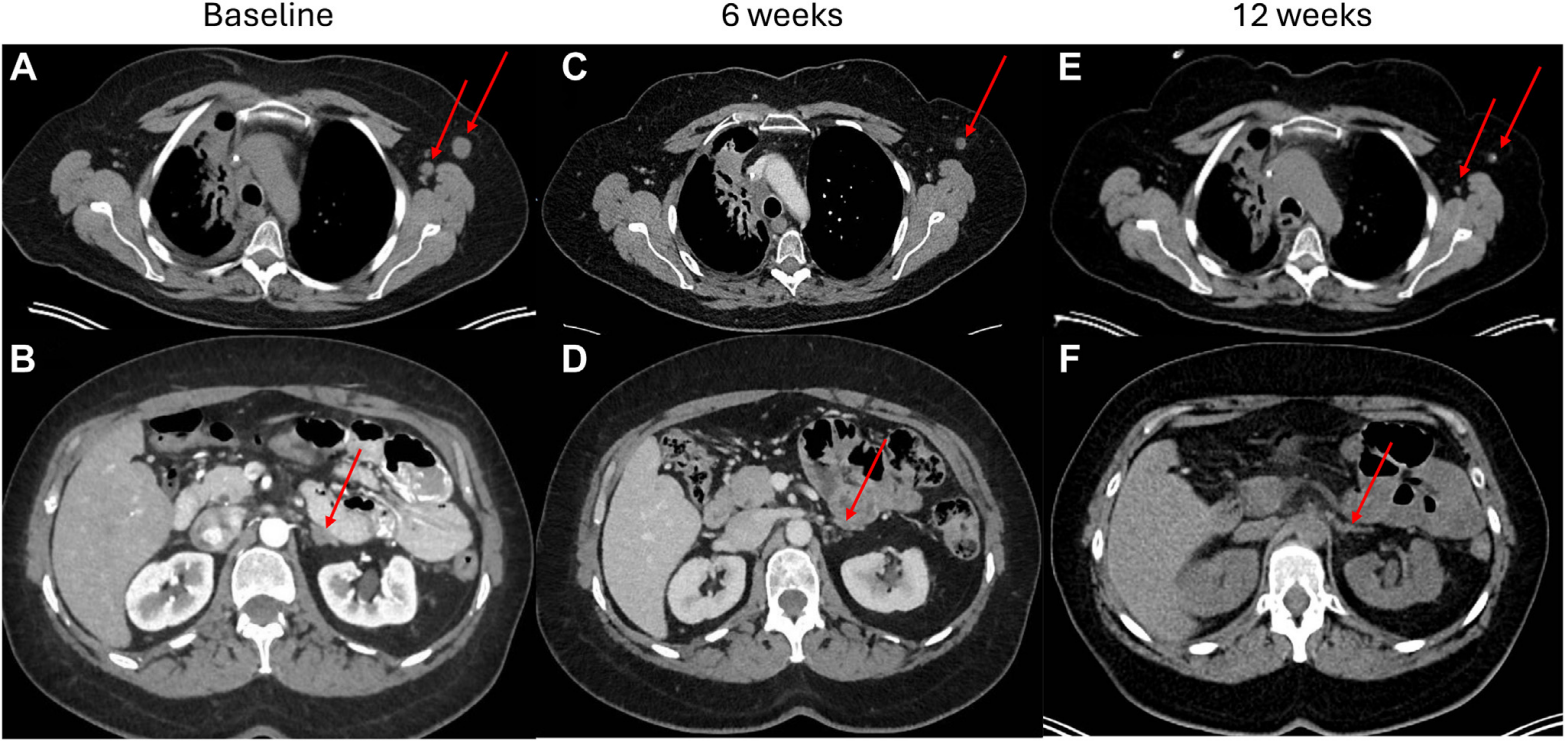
Baseline, 6-week, and 12-week imaging of key lesions revealing response. (*A*) Baseline, CT component of PET/Dotatate, enlarged left axillary lymph nodes; (*B*) baseline, CT abdomen, left adrenal metastasis; (*C*) 6 weeks, CT chest, left axillary node; (*D*) 6 weeks, CT abdomen, left adrenal metastasis; (*E*) 12 weeks, CT component of PET/Dotatate, left axillary lymph nodes; (*F*) 12 weeks, CT component of PET/Dotatate, left adrenal metastasis. CT, computed tomography; PET, positron emission tomography.
